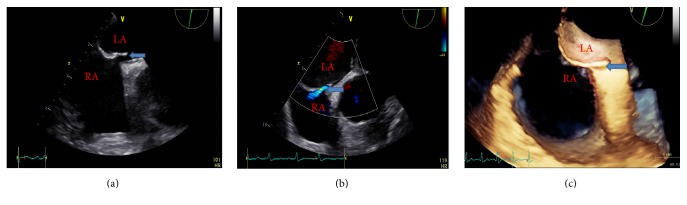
Transesophageal echocardiography demonstrating the interatrial septum. (a) Two-dimensional transesophageal echocardiography showing a “slit-like” communication between the left and right atria, which was diagnosed as a PFO. (b) Color flow mapping of the spontaneous PFO left-to-right shunt. (c) Detection of PFO by real-time three-dimensional transesophageal echocardiography. The tunnel-like configuration between the septum primum and the septum secundum, which indicates the presence of a PFO (blue arrow). LA = left atrium; RA = right atrium.
